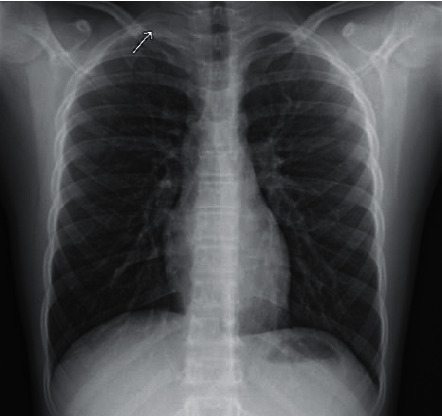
Chest radiograph showing a rounded density (32 mm × 22 mm) at the right lung apex.
